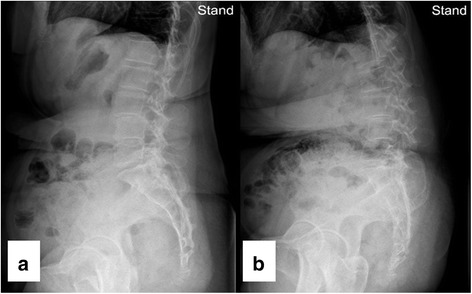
X-ray images of the lateral view of the spine on neutral standing position a one month before and b one month after administration of levodopa demonstrate progression of camptocormia despite medication
